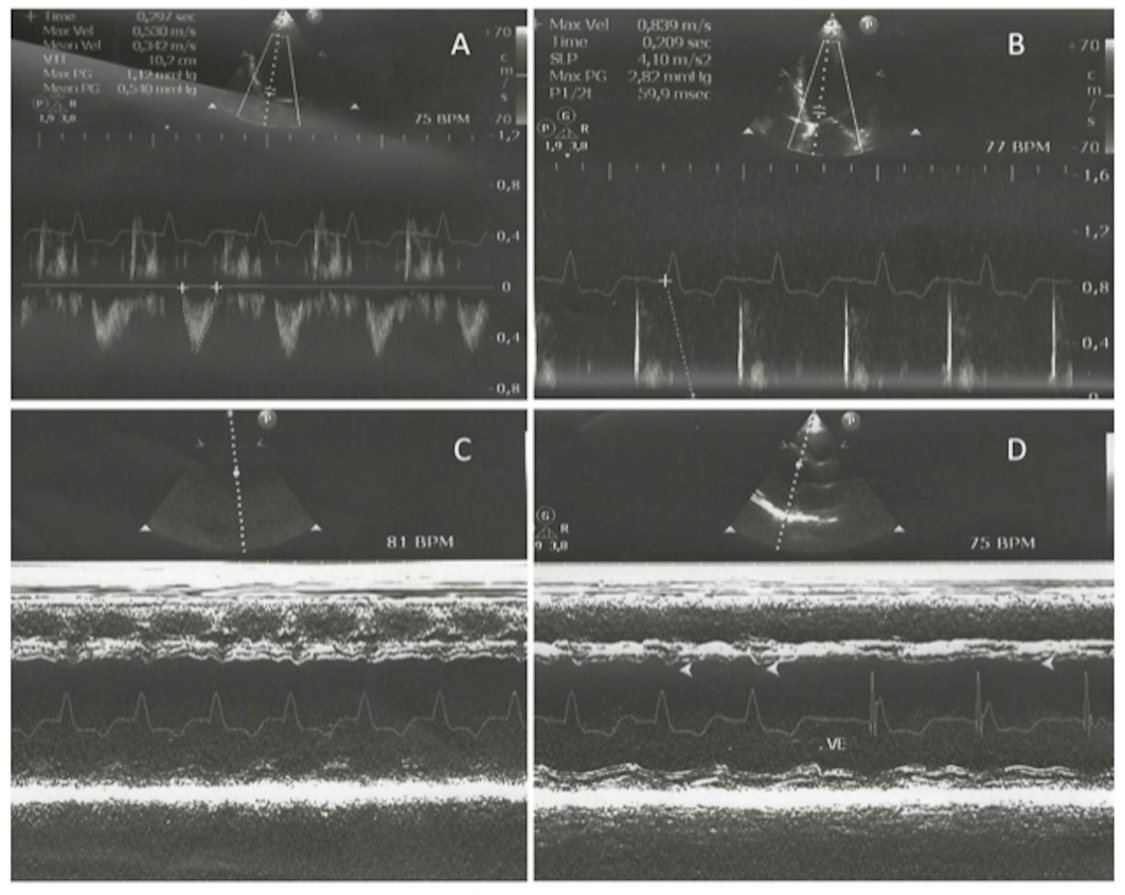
Echo Parameters Echocardiographic measures of velocity time integral (VTI) obtained in the LV outflow tract by pulsed Doppler (A) and of the pre-ejection period of the left ventricle (PEP) measured from the onset of the QRS complex to the beginning to the aortic ejection (B). M-mode tracings (C and D) showing abnormal septum motion (arrows) in the presence of left bundle-branch block, which disappeared after biventricular pacing.
